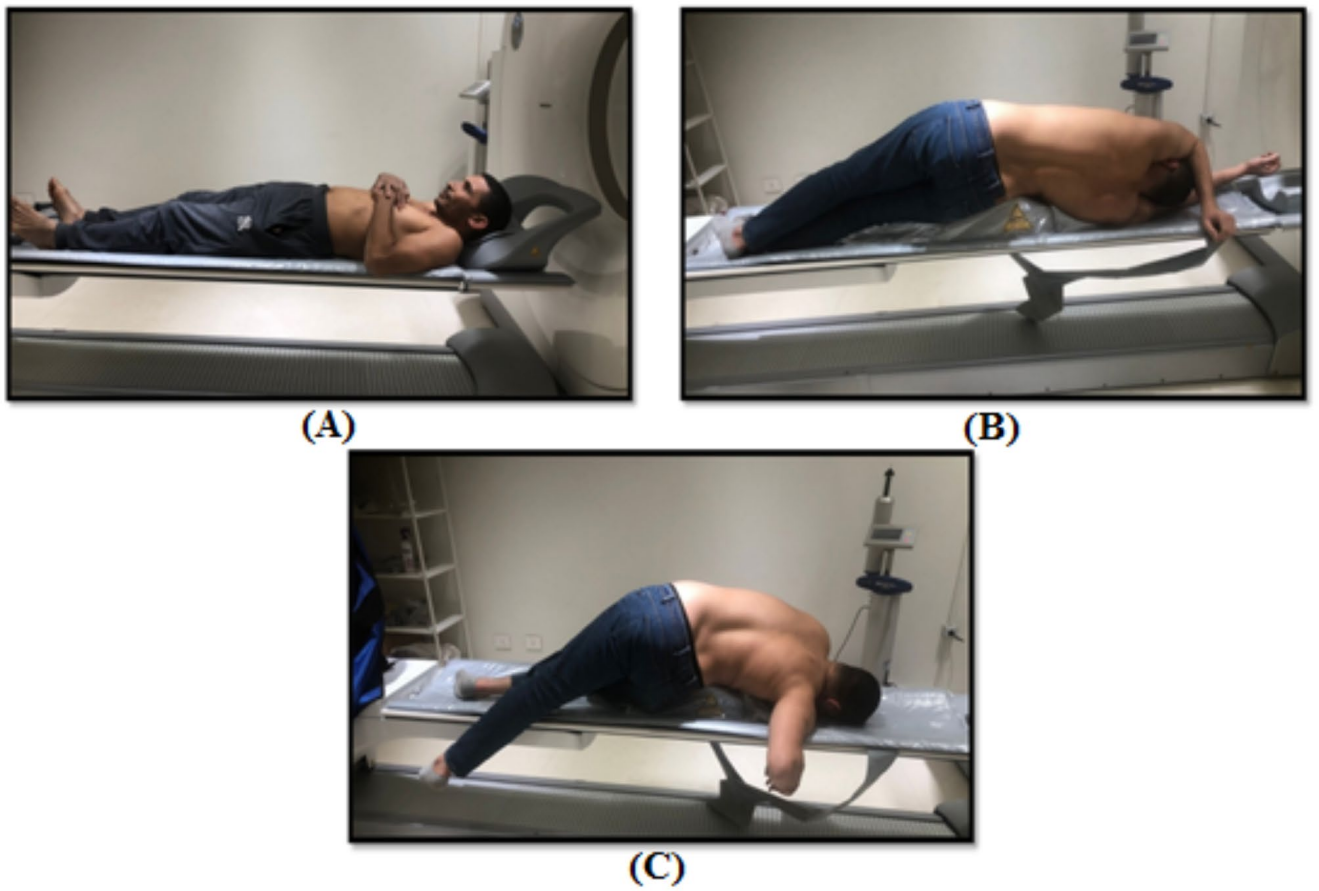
Body positions during 3D-CT scan imaging of asymptomatic individuals: (A) Conventional neutral supine position. (B) Lumbar side bending toward the pain-free side. (C) Modified reversed contralateral axial rotation (MRCAR) position; lumbar side bending and rotation toward the pain-free side
